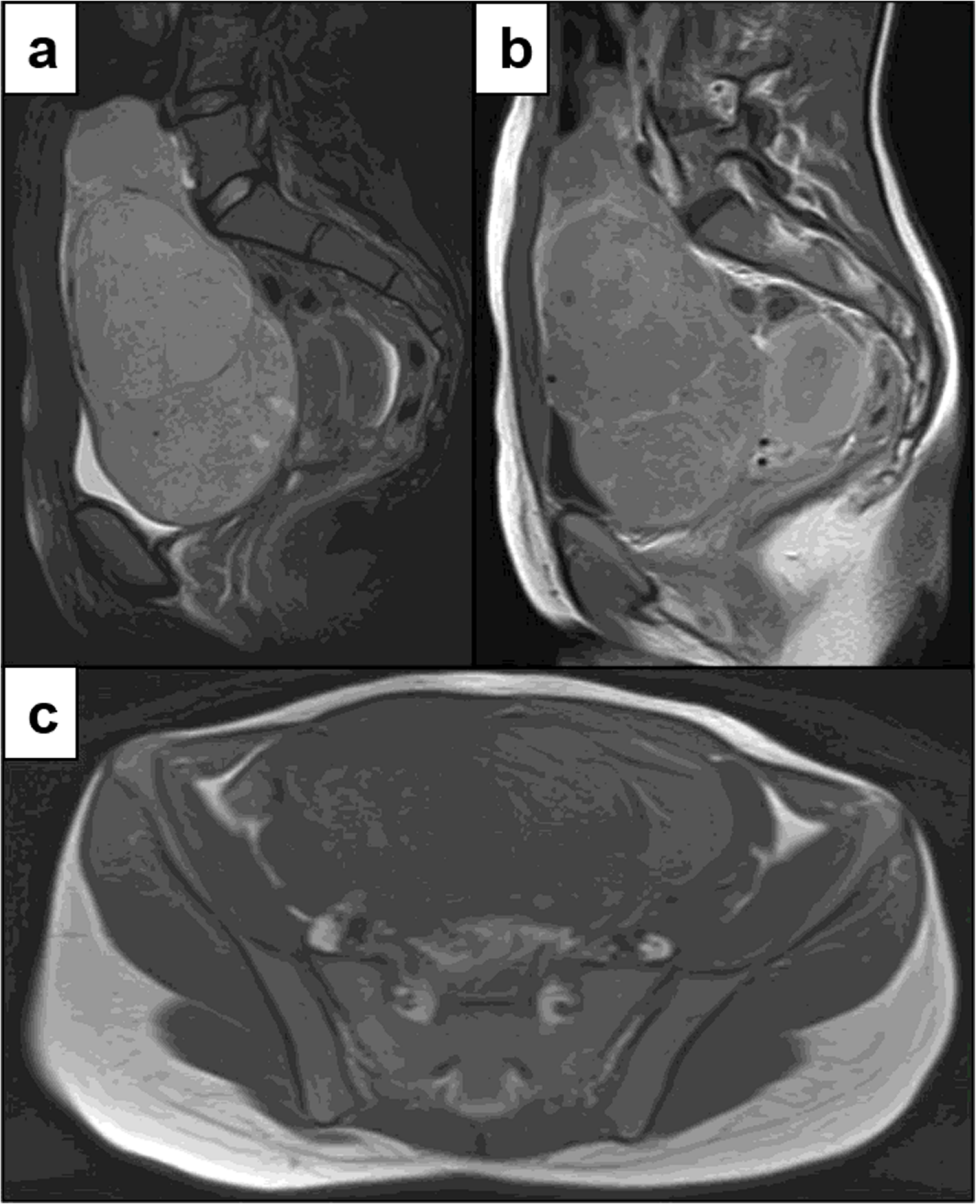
Ovarian dysgerminoma in a 20-year-old woman. Sagittal Fat Sat T2w (a), sagittal contrast-enhanced T1w (b), axial T1w (c) MR images show a capsulated giant multilobulated mass displacing both bladder and uterus, isointense to the muscle tissue on T1w, with multiple lobules hyper-intense on T2w, separated by thin, regular, hypo-intense septa with avid enhancement after Gd administration. No papillary projection, parietal irregularities or calcifications are noted
